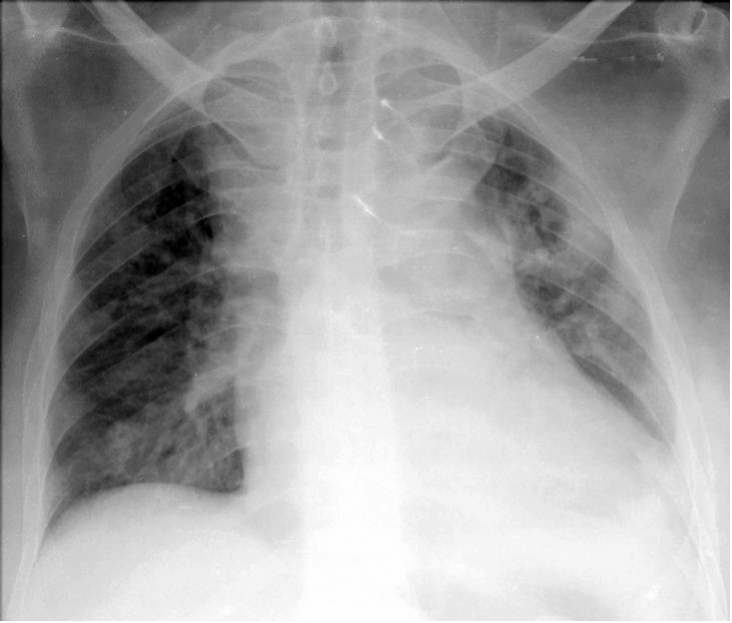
Chest radiograph showing thick walled cavity left upper lobe
